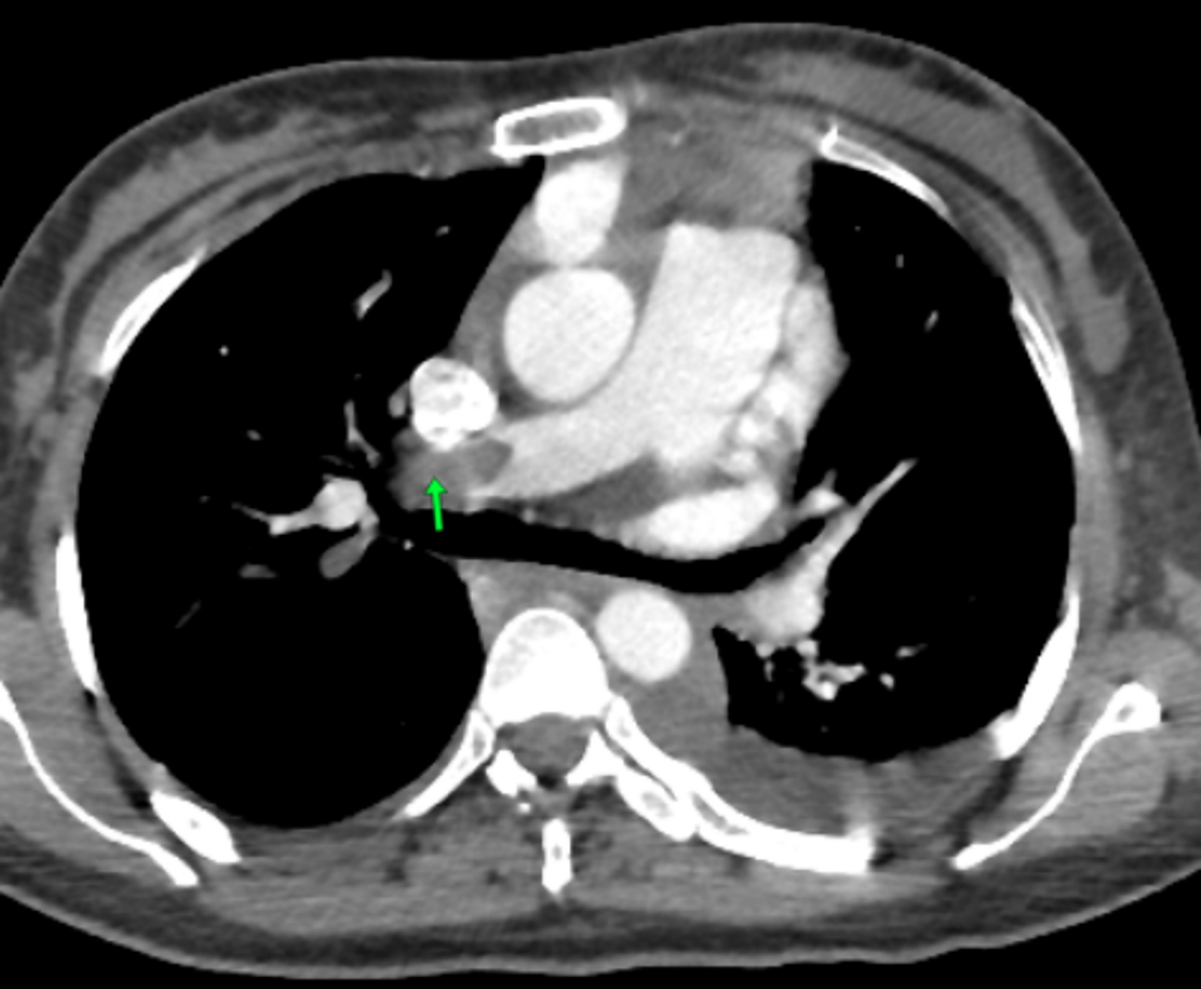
Chest CT angiogram shows embolic occlusion of the right pulmonary artery (arrow) CT: computed tomography
